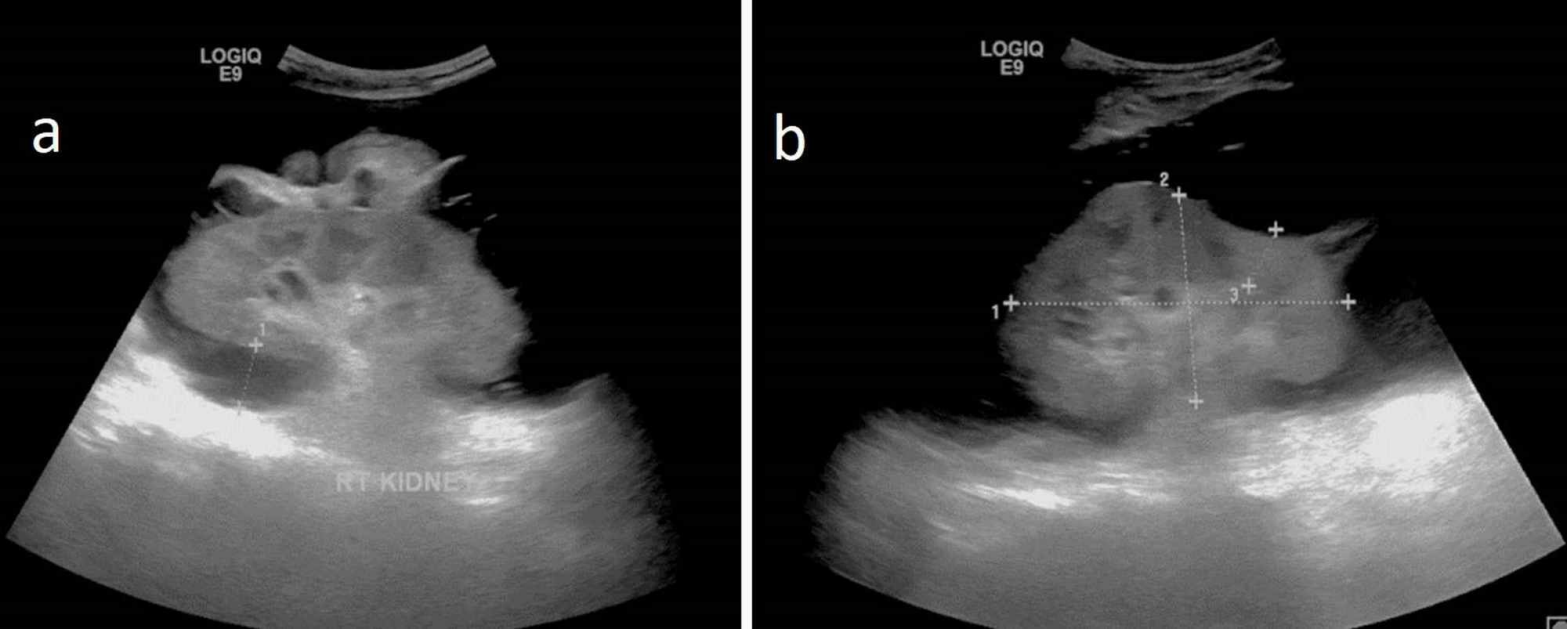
43-year-old male – a case of Budd-Chiari syndrome with bilateral perinephric renal lymphangiectasia Greyscale ultrasound in the oblique sagittal plane of both kidneys (a: right kidney, b: left kidney) showing anechoic collection with internal septations in the perinephric region on both sides
